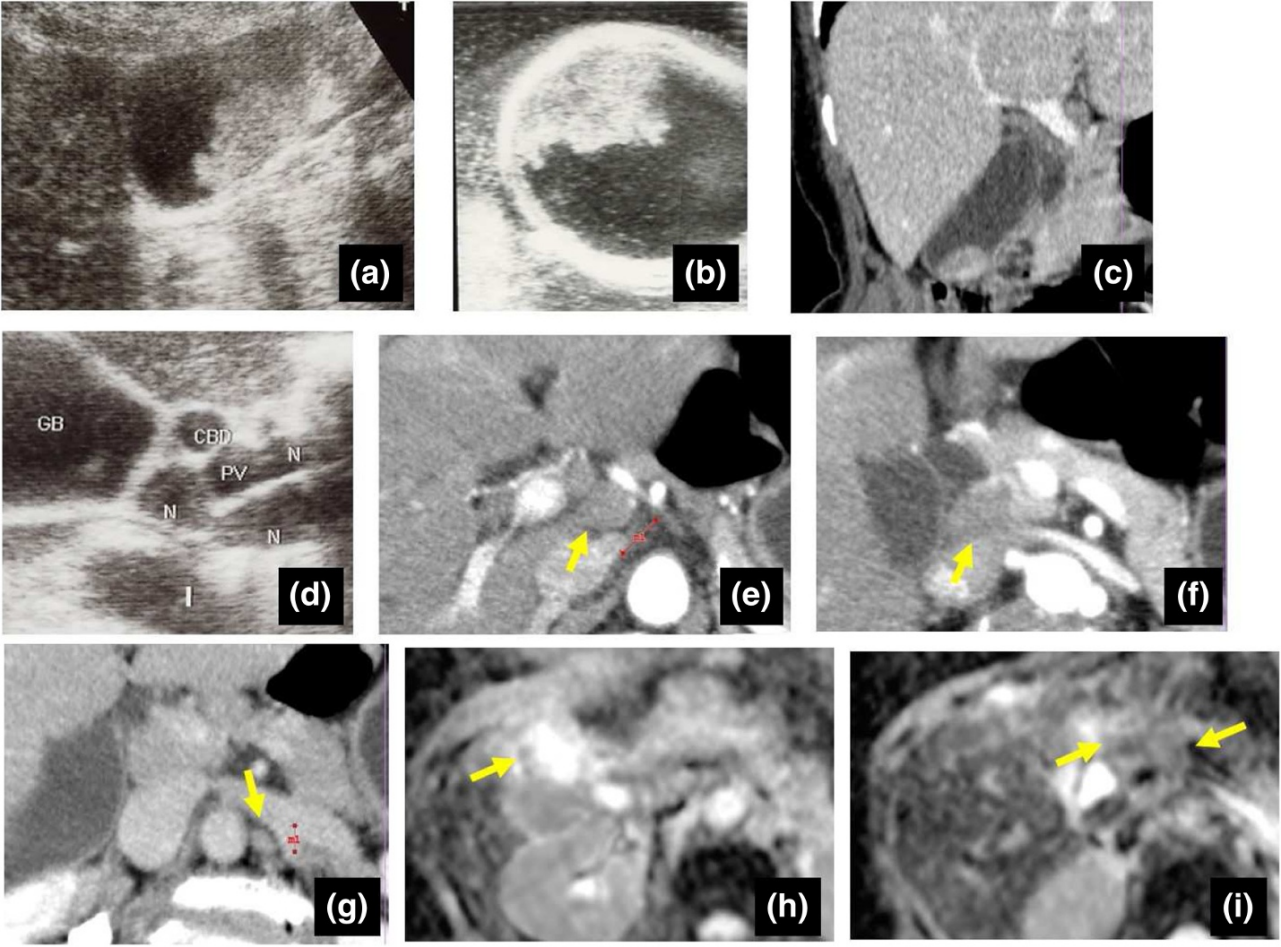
Pre- and intraoperative images. a-c) Ultrasonography (US) shows a highly echoic mass in the gallbladder. The size was φ30 mm. The widespread lesion showed irregular, gross progression, corresponding to gallbladder cancer. Because the outer layer of the gallbladder was clearly preserved (especially on intraoperative US), indicating Type B, tumor depth was estimated as extending to the muscularis propria. Computed tomography (CT) also shows a mass in the gallbladder, with no invasion to other organs. d) US shows multiple swollen lymph nodes in the hepatoduodenal ligament. e-g) CT shows swelling of not only lymph nodes at the hepatoduodenal ligament, but also para-aortic lymph nodes. h,i) Diffusion-weighted imaging (DWI) shows signal hyperintensity for the gallbladder mass, but signal hypointensity in all detected swollen lymph nodes.
